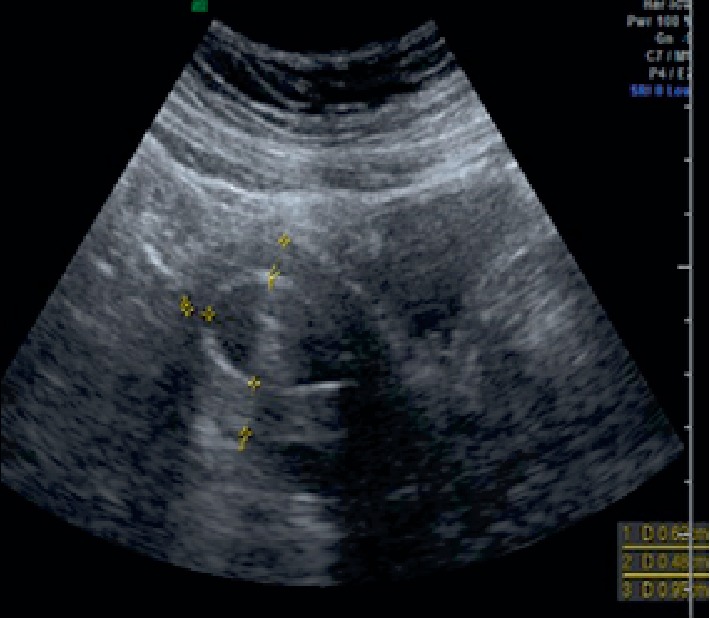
Transabdominal ultrasound was used to measure the balloon size.
